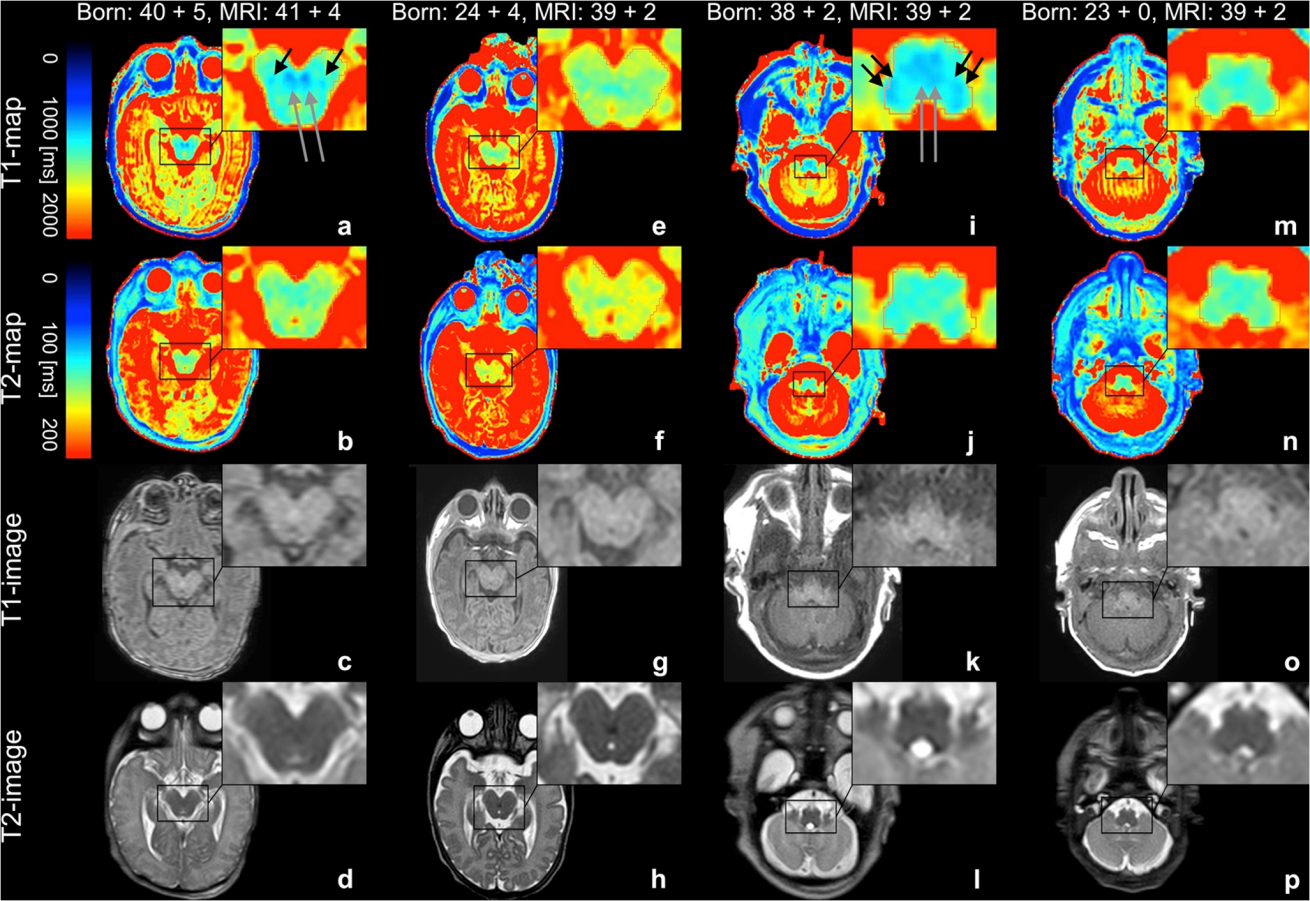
Quantitative T1-/T2-maps are shown in the upper row. T1- and T2-relaxation constants are represented by the colored bars. Conventional T1-/T2-weighted images are shown in the bottom row. a–d and i–l Data from a term-born infant. e–h and m–p Data from a former premature infant. The quantitative T1-map of the midbrain of the term-born neonate (a) shows a distinct myelination of the brachium conjunctivum (long arrows) and medial lemniscus (short arrows). A corresponding signal is far less detectable on the T2-map (b) and completely absent in the preterm neonate (e, f). Conventional MR images of the midbrain are shown for comparison (c, d, g, h). The quantitative T1-map of the medulla oblongata of the term-born neonate (i) shows a distinct myelination of the inferior cerebellar peduncle (short double arrows) and medial lemniscus (long double arrow). A corresponding signal is far less detectable on the T2-map (j) and completely absent in the preterm neonate (m, n). Conventional MR images of the medulla oblongata are shown for comparison (k, l, o, p)
